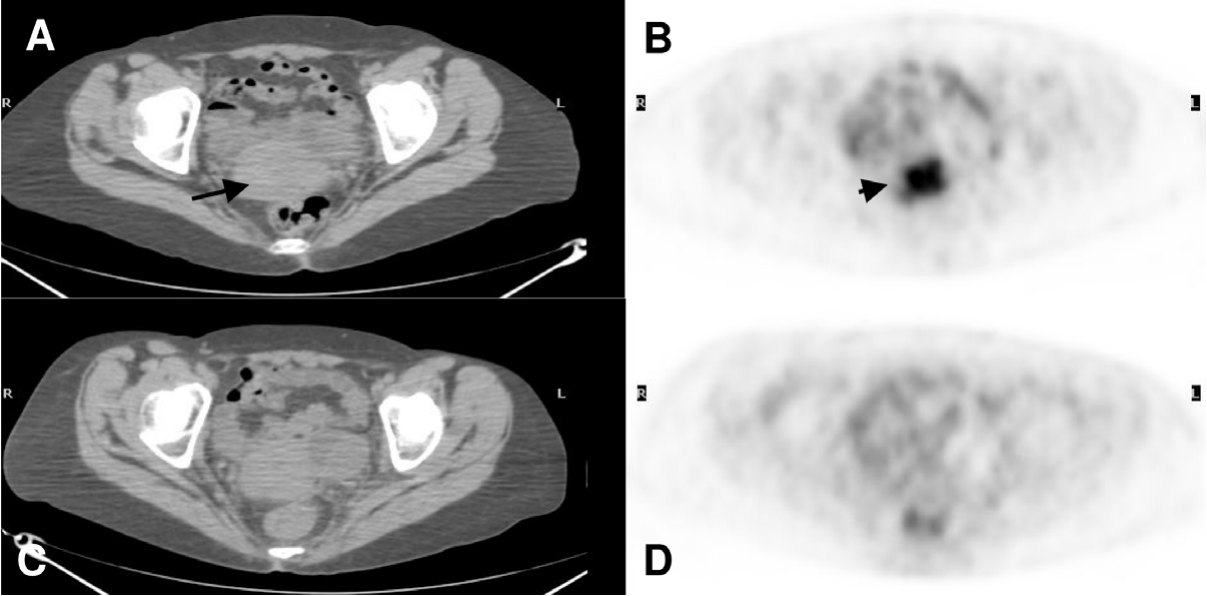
(A) Axial computed tomography and (B) axial positron emission tomography images of pre-treatment PET/CT scan showed an intense FDG focus in the uterine cervix (short arrow) with soft tissue fullness on CT (long arrow). (C) Axial CT and (D) axial PET images of post-treatment PET/CT, three months after completion of chemotherapy, showed resolution of previously FDG avid cervical lesion.
